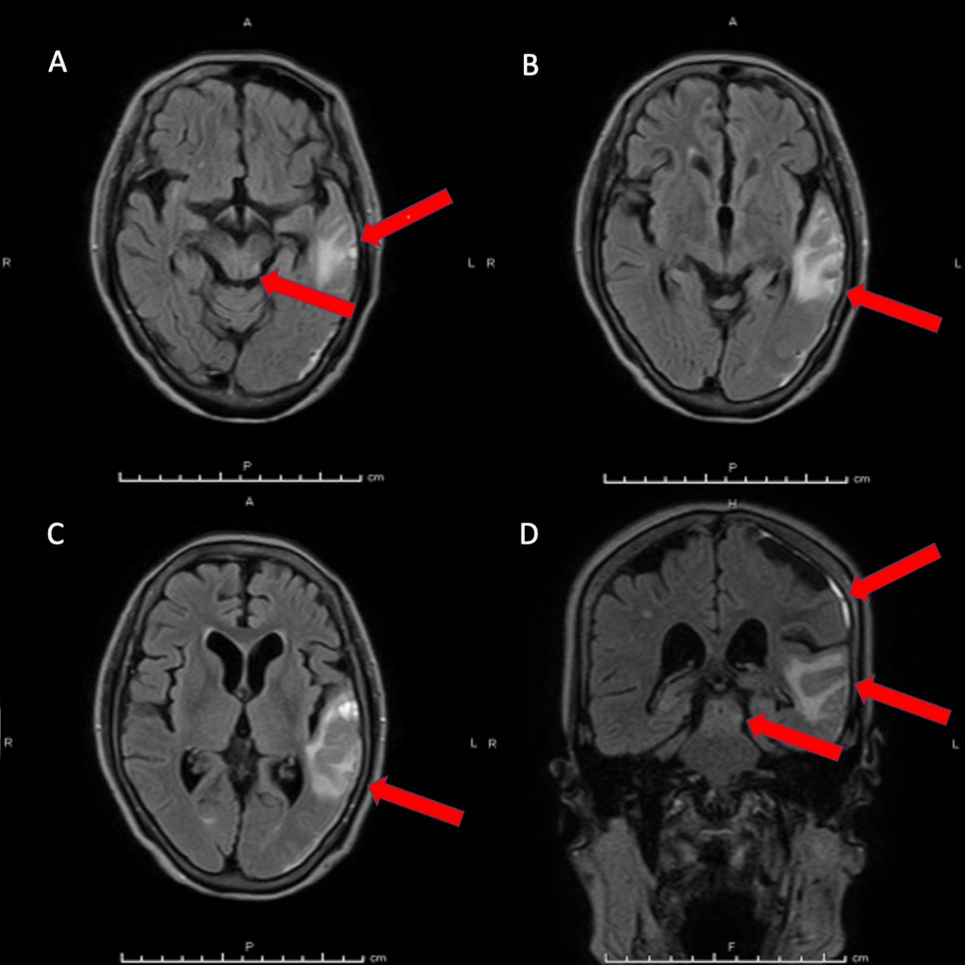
Magnetic resonance imaging (MRI) findings on hospital day 13. Signal hyperintensity on fluid-attenuated inversion recovery (FLAIR) is apparent in the midbrain (A) and left temporal lobe (B, C). Brain contusion is also seen in the left temporal lobe. Coronal-view FLAIR shows contusion in the midbrain and left temporal lobe and subdural hemorrhage in the left temporal lobe (D). Red arrows show the respective lesions
